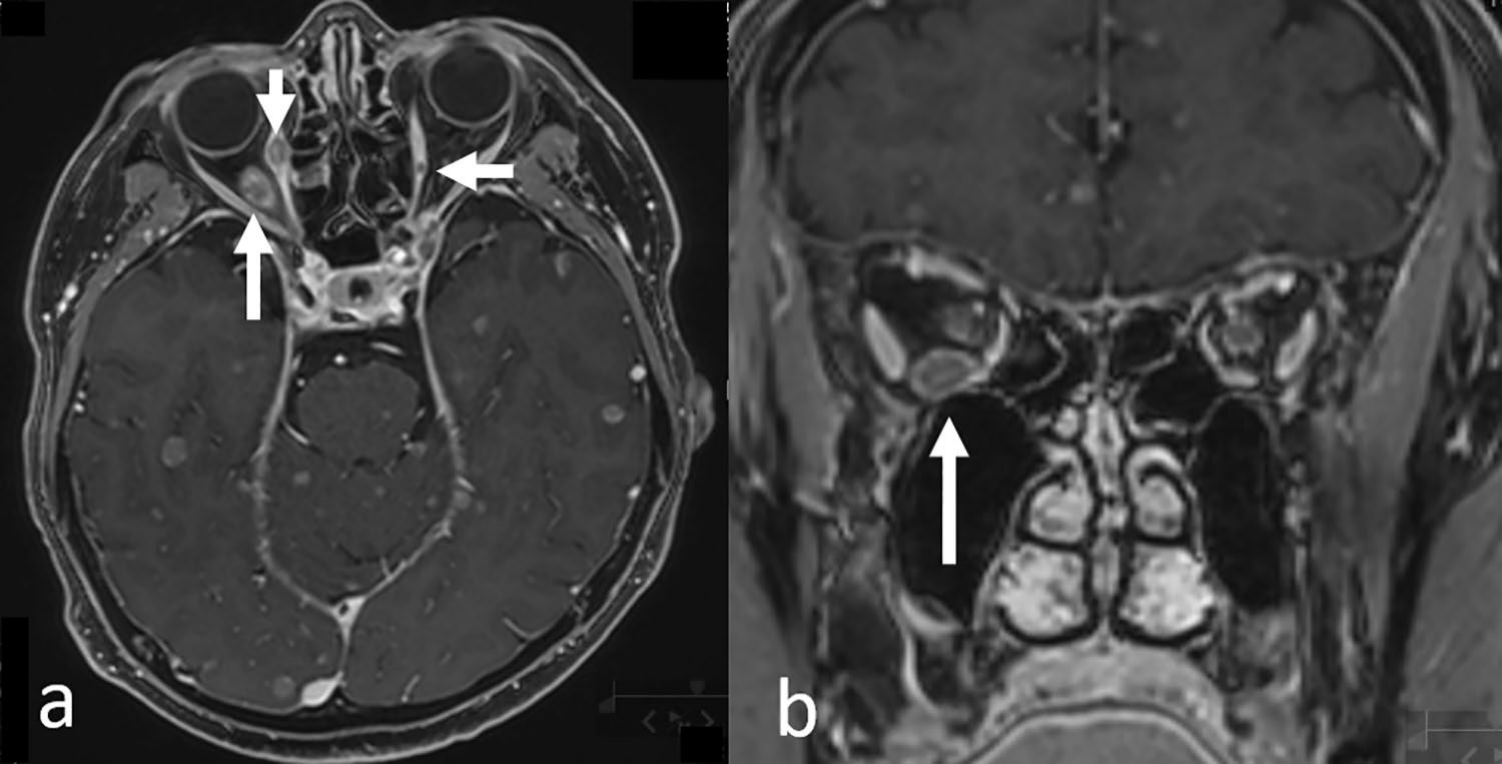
Multiple well-defined extraocular muscle metastases in a patient with small cell carcinoma of the prostate. Axial a and coronal b reconstructed contrast-enhanced 3D fat-suppressed T1-weighted images show multiple well-defined nodular tumors as less contrast-enhanced masses (arrows) in the extraocular muscles
